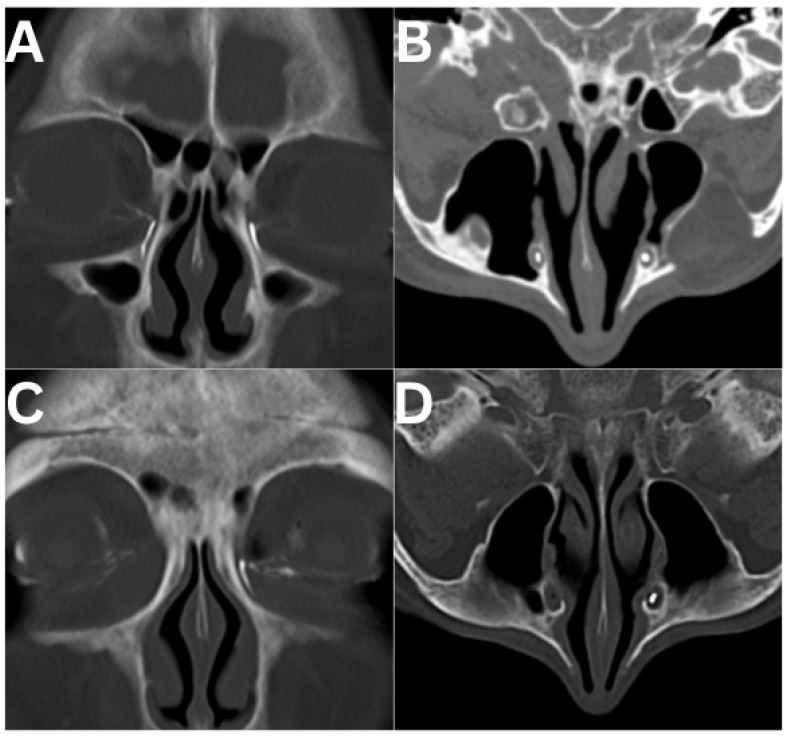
CT-dacryocystography findings in patients with chronic epiphora. (A,B) Normal nasolacrimal duct patency with continuous passage of contrast material into the nasal cavity. (C,D) Nasolacrimal duct obstruction demonstrating accumulation of contrast material with absence of distal flow.
